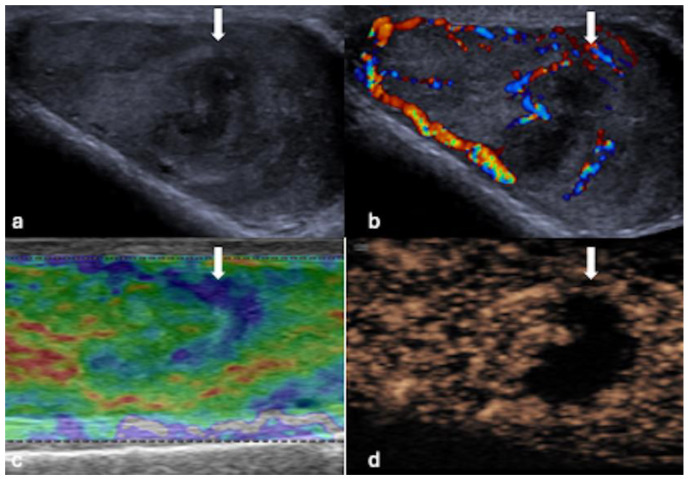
MPUS of a segmental infarction. (a) Greyscale US shows a lesion (white arrow) with a low echogenic centre and surrounding high echogenicity. (b) CDUS indicates that no colour Doppler signal is present within the lesion (white arrow). (c) On SE, this lesion (white arrow) demonstrates a predominantly green signal consistent with a “soft” lesion. (d) CEUS conclusively demonstrates the absence of enhancement within the central aspects of the lesion (white arrow).
